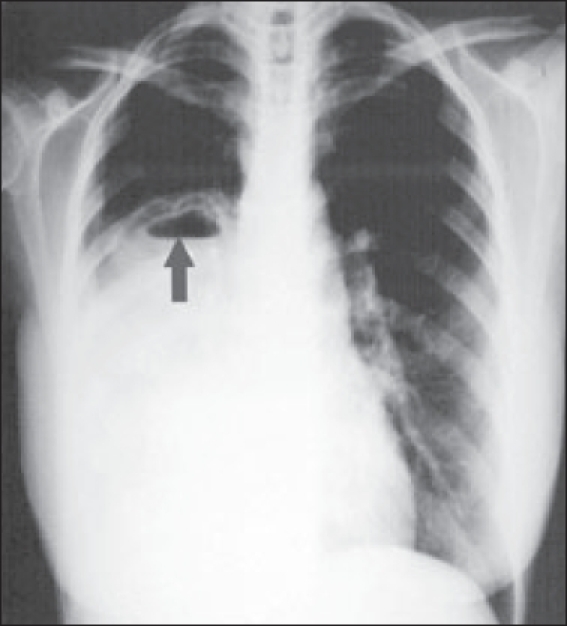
Chest radiograph at initial presentation showing uniform opacification of right lower zone, which mimicked pleural effusion and obscured the underlying cyst with air fluid level (gray arrow)
